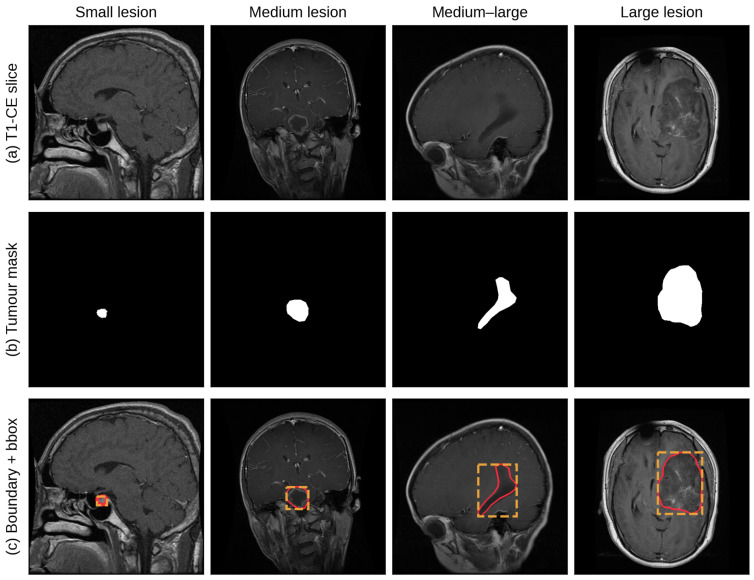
Representative contrast-enhanced T1-weighted MRI slices from the Figshare/Cheng dataset. The examples cover small, medium, and large tumour regions across axial, coronal, and sagittal planes. (a) Input T1-CE slice; (b) binary tumour mask; (c) tumour boundary and bounding box overlaid on the input slice, where the red solid line denotes the delineated tumour contour and the orange dashed rectangle denotes the corresponding bounding box.
